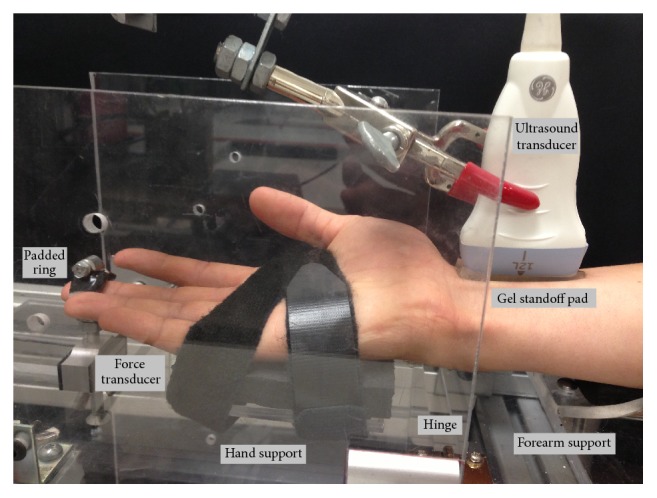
Experimental setup indicating hand and wrist posture, padded ring attached to force transducer, location of the hinge linking the forearm support and hand support, and the location of the ultrasound transducer (probe).
